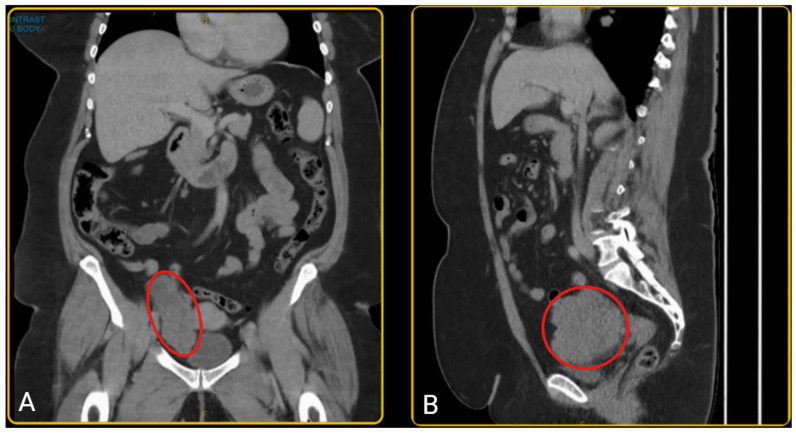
(A) The coronal view of the pelvic CT scan; (B) the sagittal view of the pelvic CT scan showing a mass in the right lower quadrant of the abdomen, anteromedial to the iliac vessels and posterior to the bladder, consistent with a right adnexal mass (outlined in red). The mass measures 103 × 98 × 50 mm, with a well-demarcated, irregular border and has a heterogenous composition with varying internal density.
